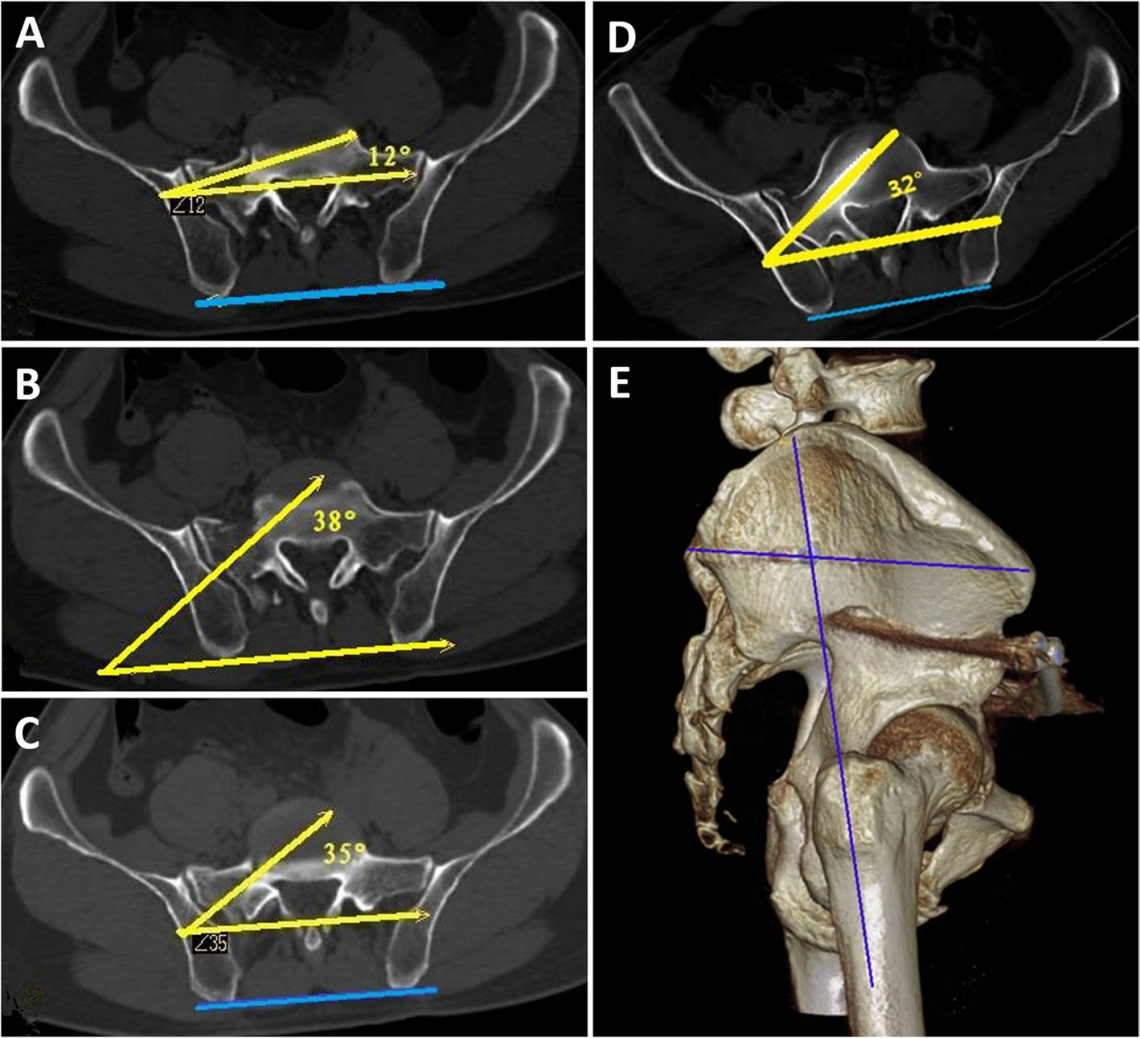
A: Mendel et al. [27] used the anterior edge of the spinal canal as the nail entry point with an angle of 12°; B: Hou et al. [28] used an anterior tilt angle of 38°; C: preoperative nail entry point angle of 35° with the posterior edge of the spinal canal; D: postoperative nail entry point angle of 32° with the posterior edge of the spinal canal; E: 3D CT reconstruction confirmed the location of the nail entry point at the intersection of the line connecting the anterior superior iliac spine and posterior inferior iliac spine with the upward extension of the femoral stem
